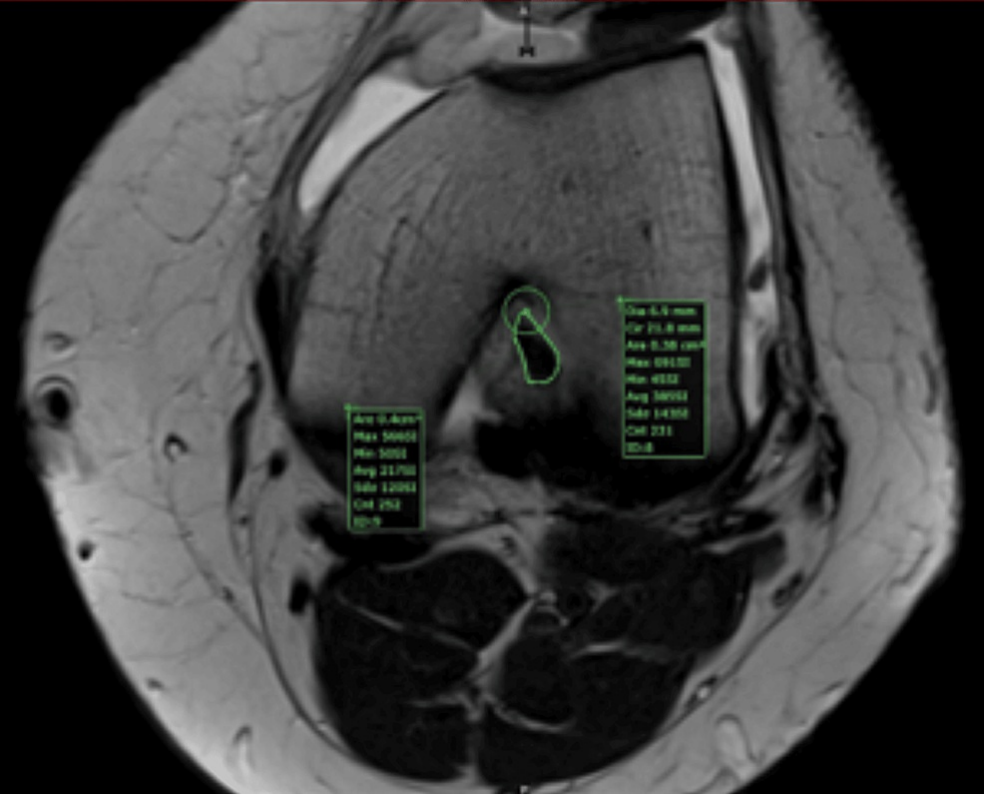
MRI measurement of the same anterior cruciate ligament (ACL) presented in Figure 7 (0.38 sq. cm area) This figure is the original work of the authors. Patient consent for the use of the image was obtained, as mentioned in the patient consent form.
